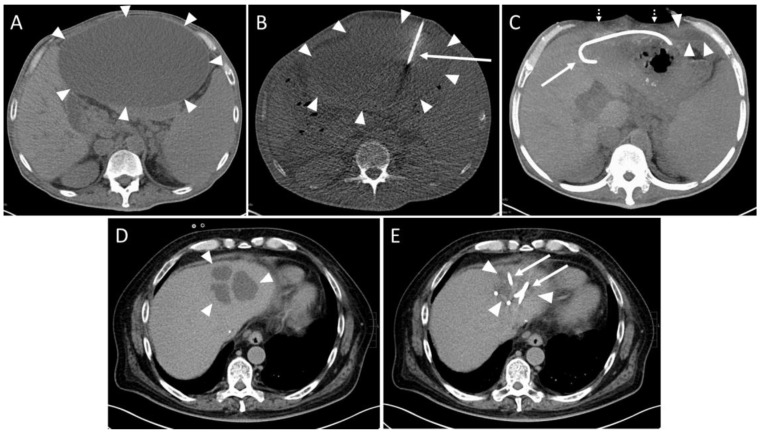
Example of a routine CT-guided drainage placement without complications. A 50-year-old man with history of liver transplantation due to alcohol-induced cirrhosis. Five weeks after surgery, an increase in transaminases and serum bilirubin was observed. Later, a palpable mass and upper abdominal pain developed. (A) CT planning scan 59 days after transplantation showed a large subcapsular fluid collection with mass effect encompassing the anterior liver margin (arrowheads). (B) CT fluoroscopy scan: An 8F drain (arrow) placement in the collection (arrowheads) via a left anterior transabdominal access using the Trocar technique was performed. (C) Maximum-intensity projection (25 mm slab thickness) of the CT control scan revealed a marked reduction in the mass effect. It was possible to aspirate 3000 mL of yellow-green fluid, resulting in almost complete drainage of the formation. Only a small residue (arrowheads) remained in the left recessus. Patient reported instant relief of symptoms (dotted arrows: collapsed abdominal wall, arrow: drain). No signs of complications such as organ penetration or bleeds are visible. Microbiological analysis revealed a sterile biloma. (D) Eight years later, the patient was readmitted due to cholangitis of the left liver lobe with several abscesses (arrowheads). (E) Again, CT-guided placement of two drains (arrows) could be successfully performed. The additional administration of pathogen specific antibiosis led to a decrease in inflammatory parameters. Arrowheads: Abscesses.
